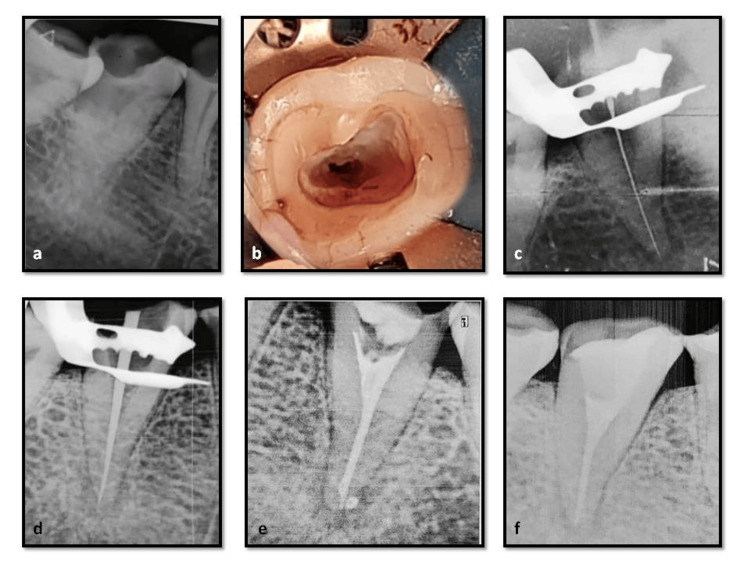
Case report-4 a) Preoperative radiograph, single conical root; b) access opening, single oval-shaped canal orifice; c) working length radiograph, single canal from orifice to the apex; d) master cone radiograph; e)obturation radiograph; f) postoperative radiograph.
